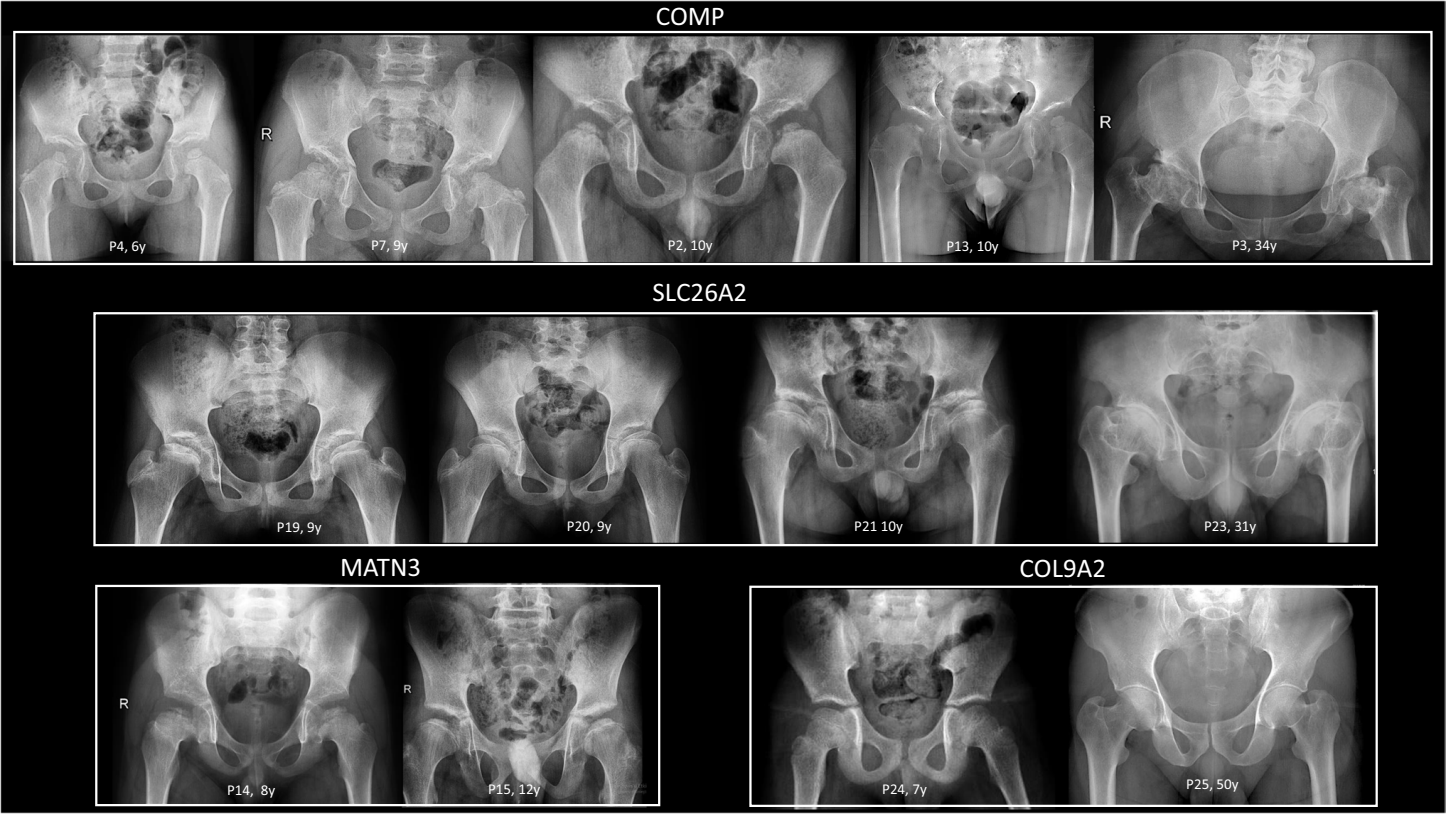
Pelvis radiographs of the patients. First row: Patients with *COMP* variants exhibit small, round epiphyses with irregular contours, except for P2, who has flattened and fragmented epiphyses (left). Coxa vara deformity with short and broad femoral necks is prominent in P7, P13, and P3. The ossified major trochanteric epiphyses are small and irregular in shape for P4 and P7. All patients present with dysplastic acetabula, and the adult patient (P3) has secondary osteoarthritis. Second row: Patients with *SLC26A2* variants show flattened epiphyses, and all have dysplastic acetabula. Coxa vara deformity, with short and broad femoral necks, is observed in all patients. Third row: Patients with *MATN3* variants have flattened epiphyses, and their femoral necks are short and broad, accompanied by mild acetabular dysplasia. The femoral epiphyses appear relatively normal in the patient with the *COL9A2* variant, while his father displays short femoral necks and coxa vara. P, patient; y, years
